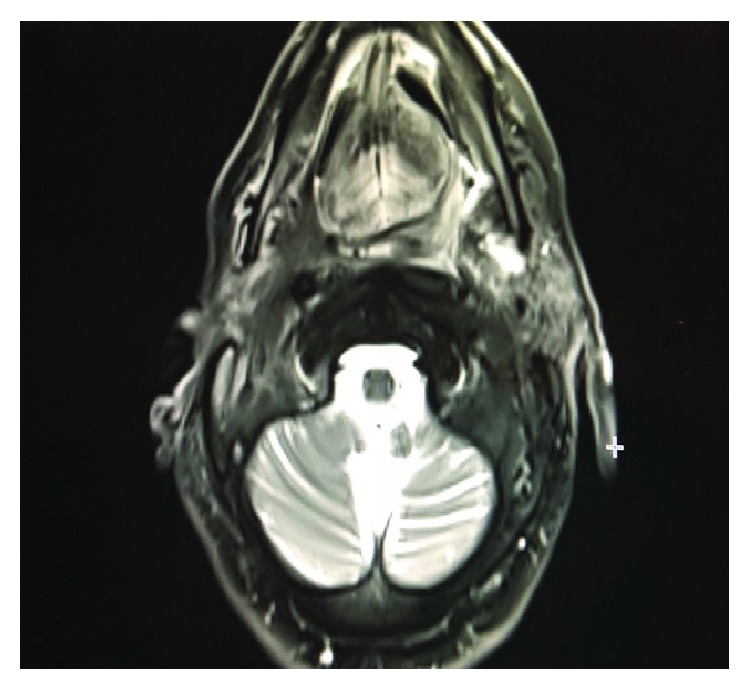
MR image of the tumor following pembrolizumab treatment: decreased tumor size revealing remission.
